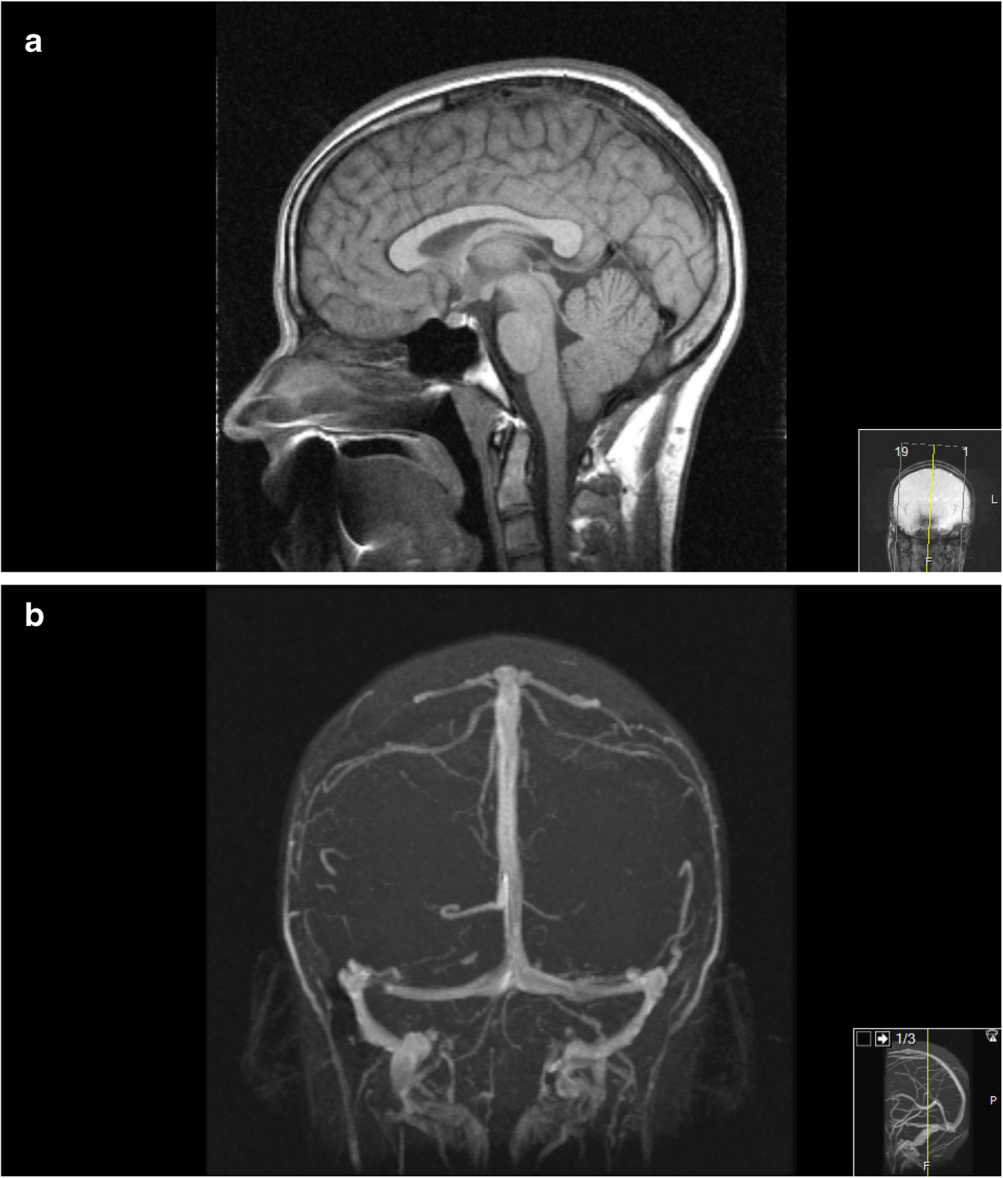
Normal MRI including venous outflow taken in 2006 during episode of shunt failure
